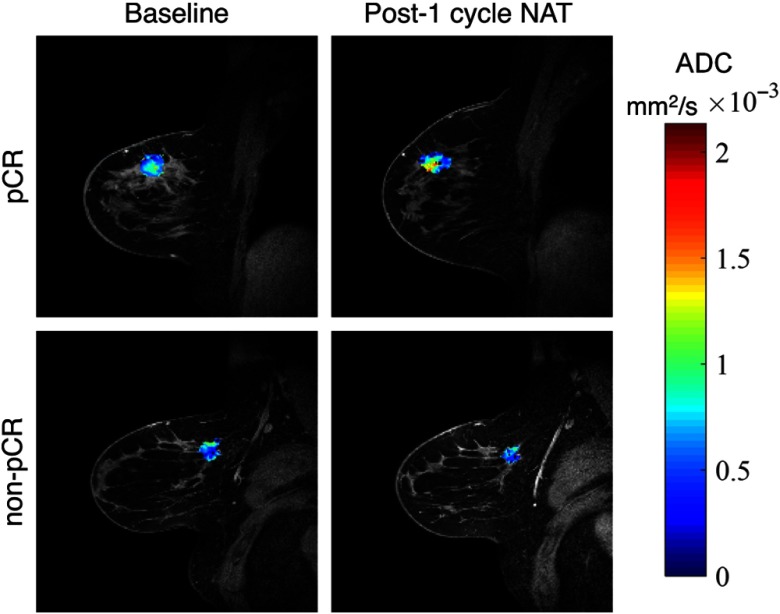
An example of DW-MRI data from a patient achieving pCR (top row) and a patient not achieving pCR (bottom row). ADC maps from the tumor region are overlaid on a high resolution anatomical image before (left column) and after the first cycle of NAT (right column). The patient who ultimately achieved pCR exhibits increased at ADC early time points within therapy, whereas the patient who did not achieve pCR does not.
